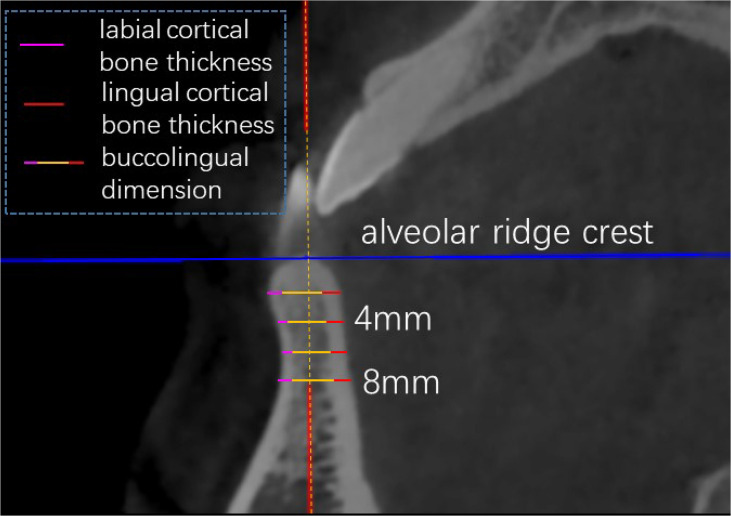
CBCT measurement: buccolingual dimension, labial cortical bone thickness and lingual cortical bone thickness in sagittal view.
